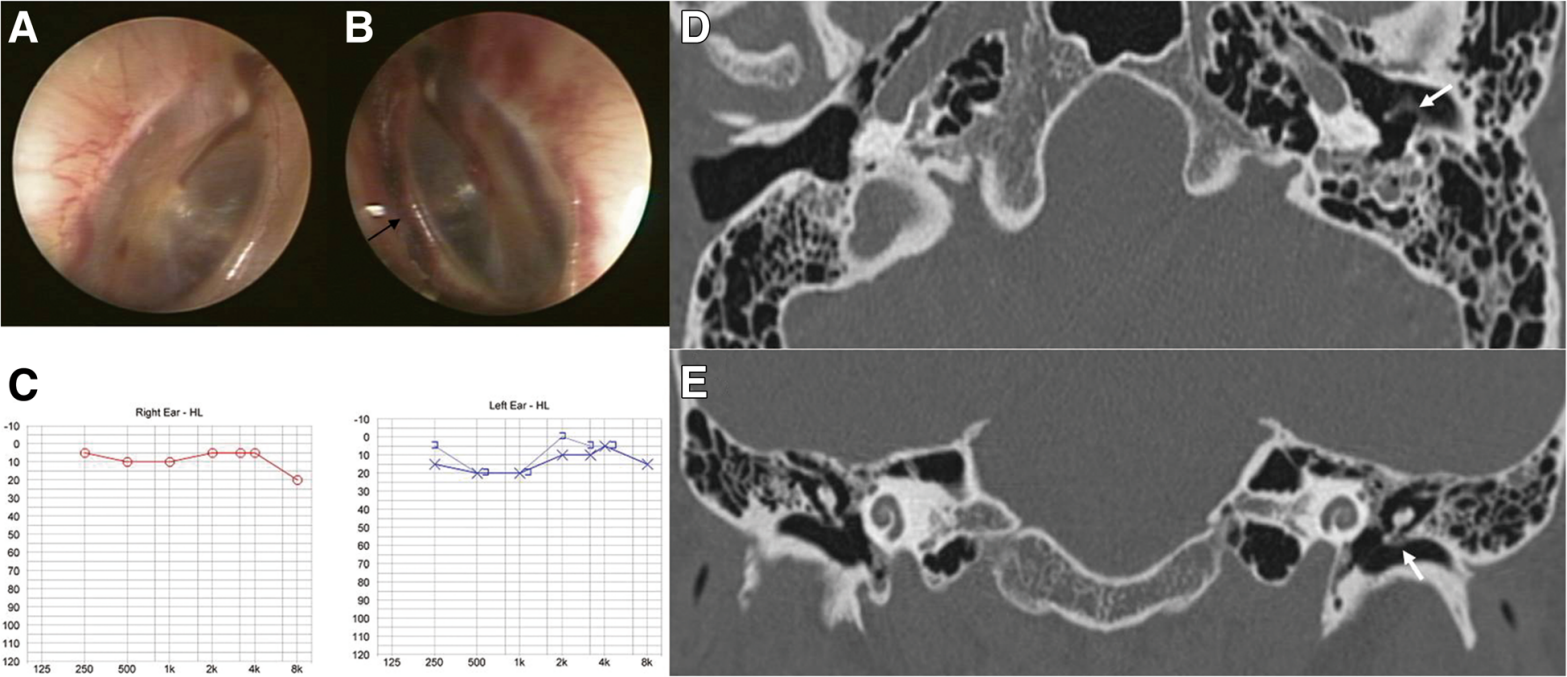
Otoendoscopic examination of patient 4 revealed minor bleeding along the manubrium of the malleus in the tympanic membrane (TM) on the right side (a), and a small hemorrhage behind the intact TM and along the line between the annular ligament and epidermis of the TM (black arrow, b). Pure tone audiometry revealed mild conductive hearing loss on the left side (c). Temporal bone computed tomography demonstrated mild thickening of the left TM on axial (d) and coronal views (e) (white arrows), suggesting iTM hemorrhage
